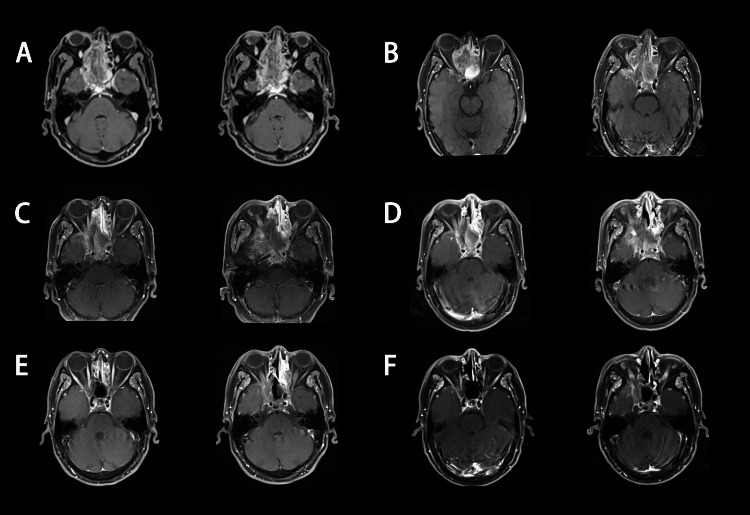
Axial T1- weighted on the administration of contrast MR imaging At the time of diagnosis (A), 18 days after surgery (B), 1.5 months after treatment (C), three months after treatment (D), seven months after treatment (E) and 13 months after treatment (F).
